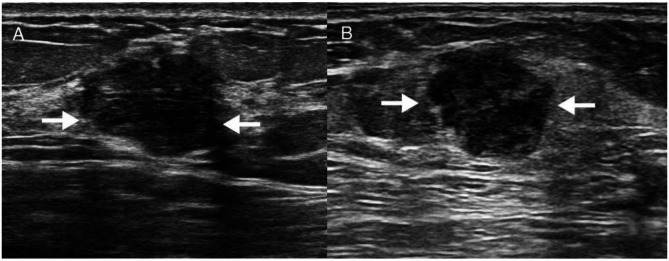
(A) Ultrasound images of patients. AFA with irregular morphology, posterior echo-enhancement and lateral shadow (white arrows); (B) TNBC with irregular morphology, posterior echo-enhancement and no lateral shadow (white arrows)
